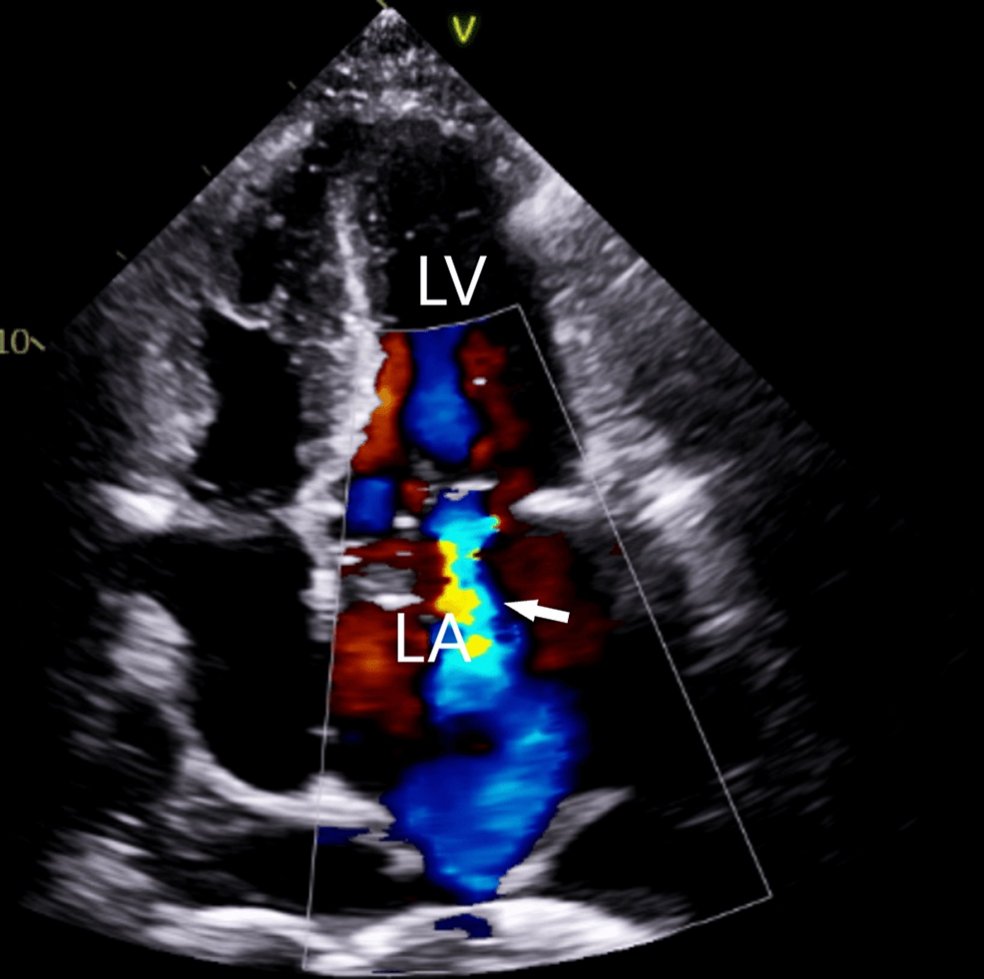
TTE showing severe mitral regurgitation (arrow). LA, left atrium; LV, left ventricle; TTE, transthoracic echocardiogram
